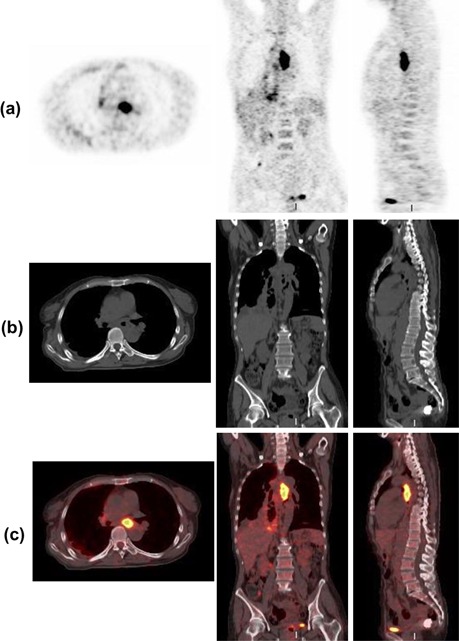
Example of PET and CT images: (a) PET; (b) CT; (c) PET/CT fused.
